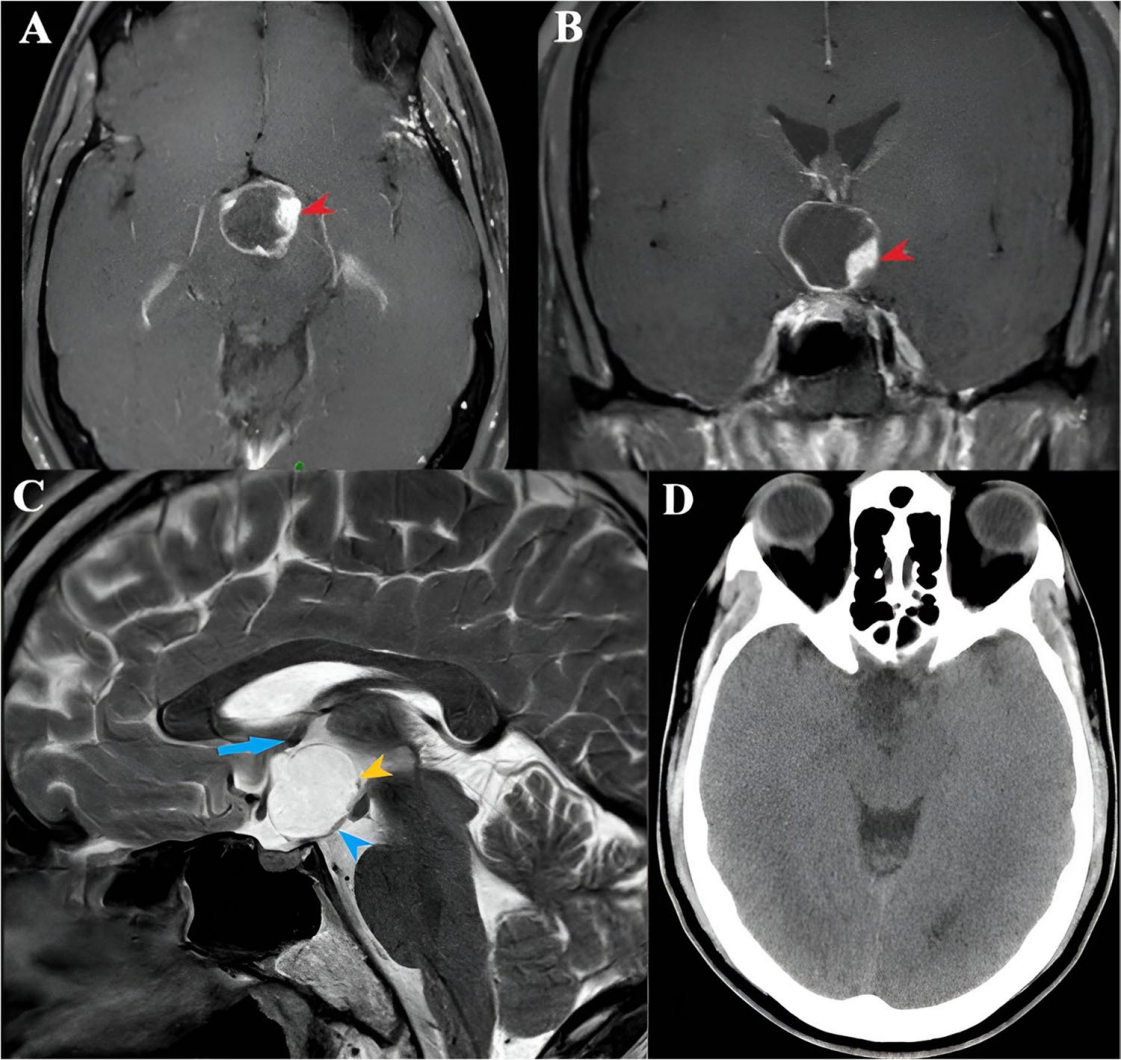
Preoperative imaging of case 1: A. and B. - Axial and coronal T1 with contrast MRI images showing the contrast enhancing nodule (red arrowhead) and cyst with irregular enhancing wall, C.- sagittal MRI images showing a cystic lesion in the suprasellar space pushing the optic chiasm (blue arrow) anteriorly and pituitary stalk (blue arrowhead) posteriorly and floor of the third ventricle (yellow arrowhead) superiorly, D. CT image show no calcifications
